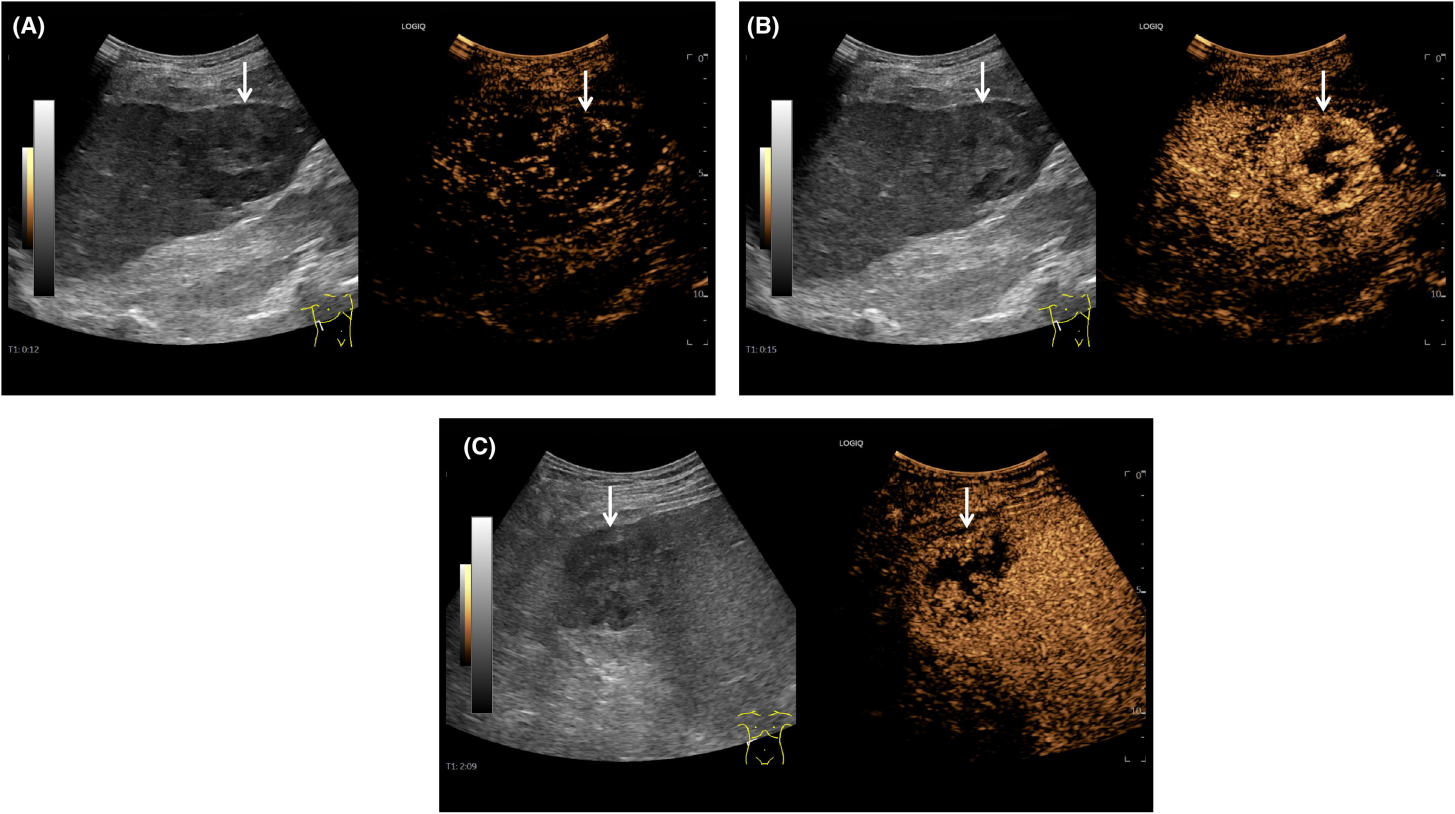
Contrast‐enhanced ultrasound of sarcomatoid hepatocellular carcinoma. (A) In the early arterial phase, the mass (arrow) rapidly enhanced beginning in the 12th second. (B) In the arterial phase, the mass (arrow) was high peripheral enhancement with no internal enhancement in the 15th second. (C) In the portal phase, the mass (arrow) was washed out in the 129th second.
